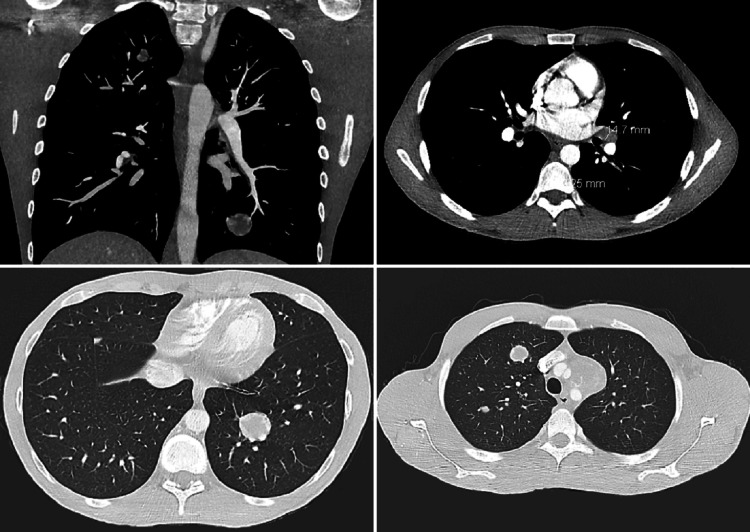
CT of the chest and mediastinum showing enlarged mediastinal lymph nodes, with the largest one measuring 3 cm, and multiple hilar lymph nodes measuring up to 1 cm.
